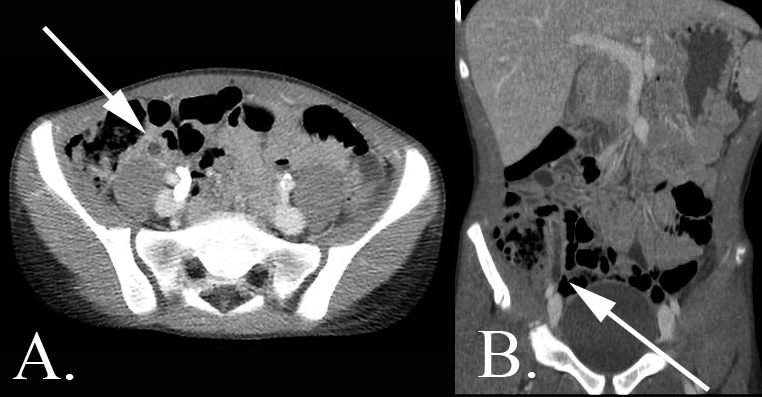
A 13-year-old male with abdominal pain who was diagnosed with uncomplicated appendicitis. (A) Axial CT and (B) coronal CT images demonstrates a dilated fluid filled appendix with a thickened and mildly hyperenhancing appendiceal wall. This appendix has a taut, elongated morphology as opposed to the redundant and coiled appearance of the normal appendix. The paucity of intraabdominal fat in this child makes the assessment of periappendiceal fat stranding challenging.
